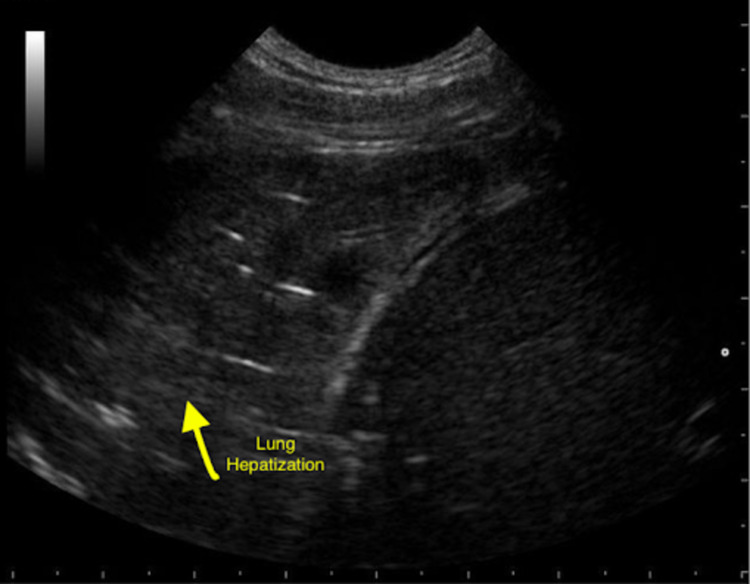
Ultrasound of the lung showing hepatization of the lung. The yellow arrow points to an area of lung hepatization representing consolidation of the lung.
